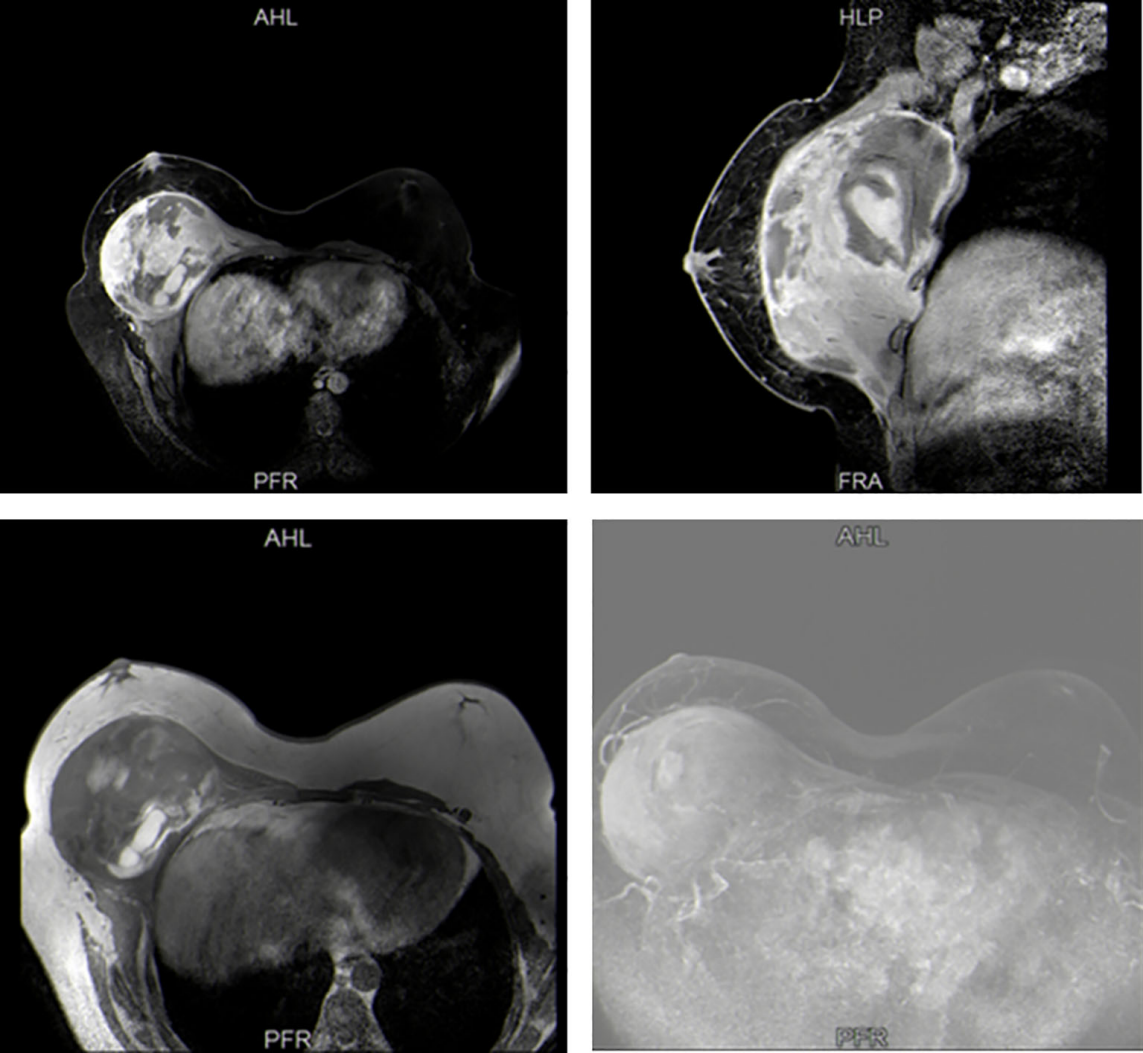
Preoperative Breast MRI.
